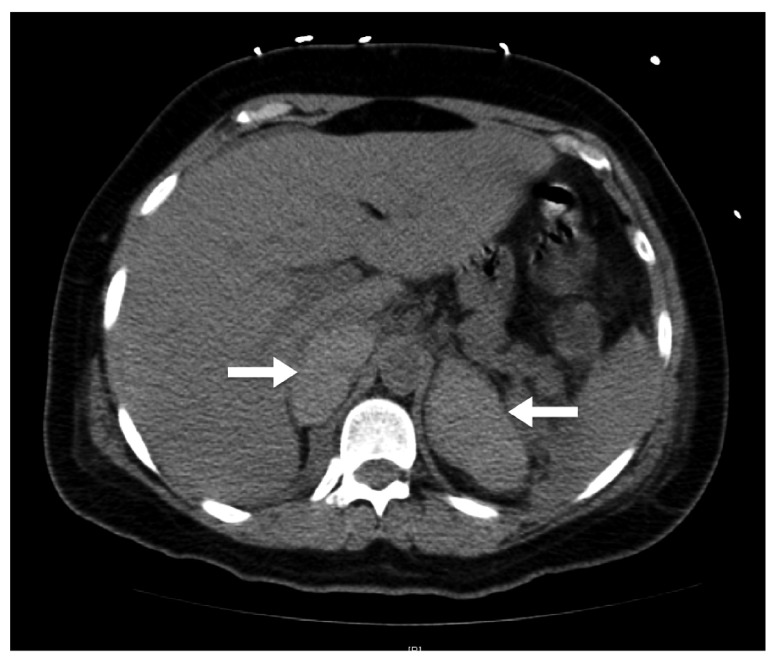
Non-contrast computed tomography scan of adrenal glands. CT scan of adrenal glands; white arrows indicate severely enlarged, hyperdense, adrenal glands bilaterally consistent with adrenal hemorrhage.
